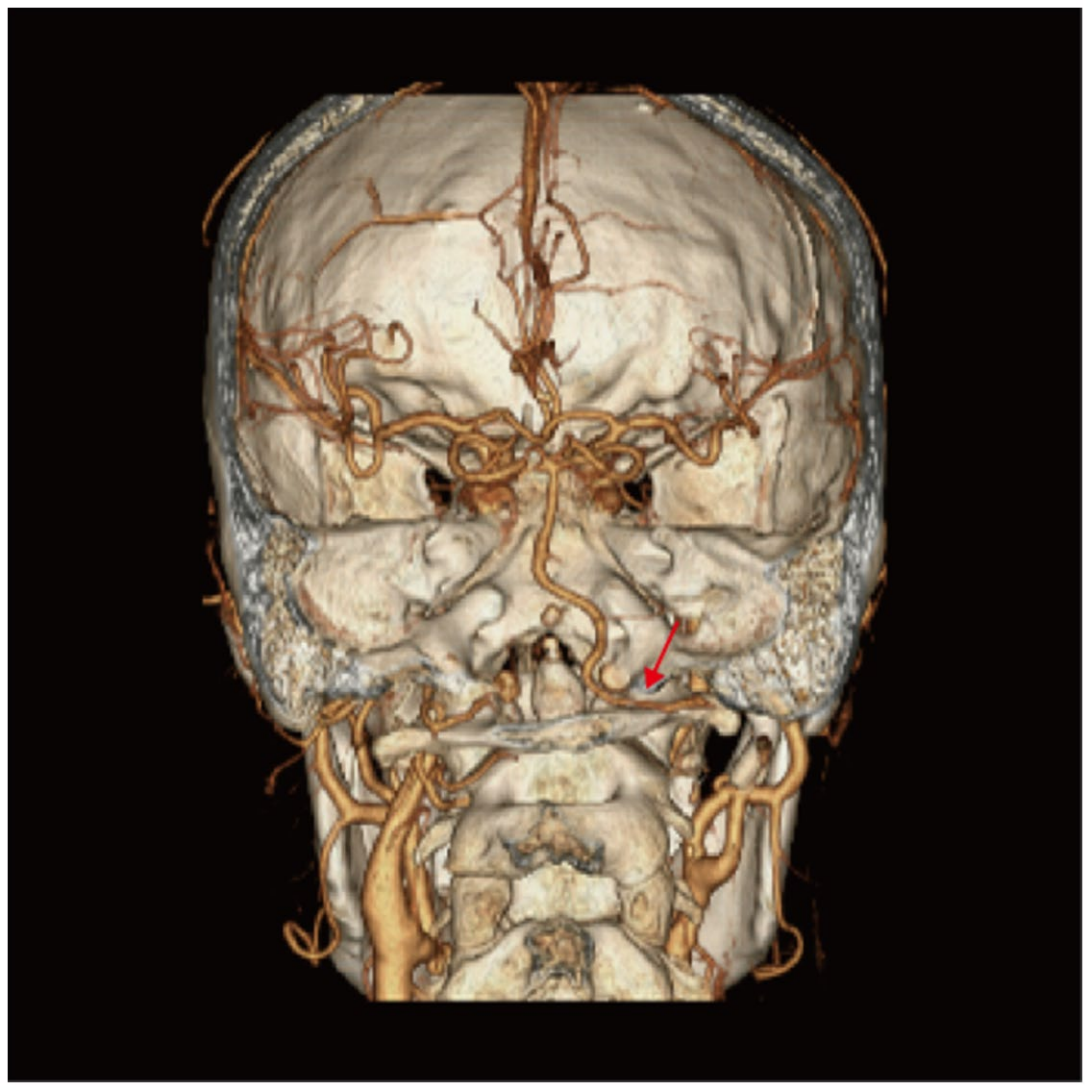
Computed tomography angiography (CTA) of the patient 1 month after persistent proatlantal inter-segmental artery (PPIA) angioplasty with flow diverter implantation. Right PPIA (arrow) aneurysmal dilatation was significantly improved compared to the pre-intervention images.
